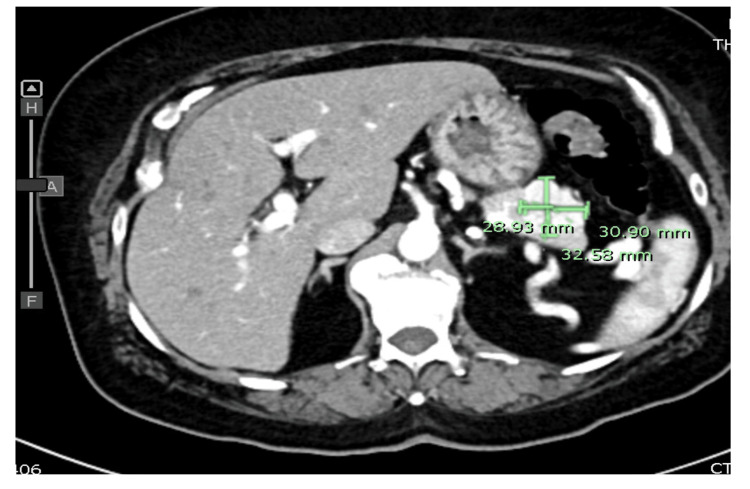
Computed tomography of the abdomen and pelvis with contrast Enhancing structure in the tail of the pancreas as described may be mildly increased in size. The appearance is suggestive of an intrapancreatic splenule.​
